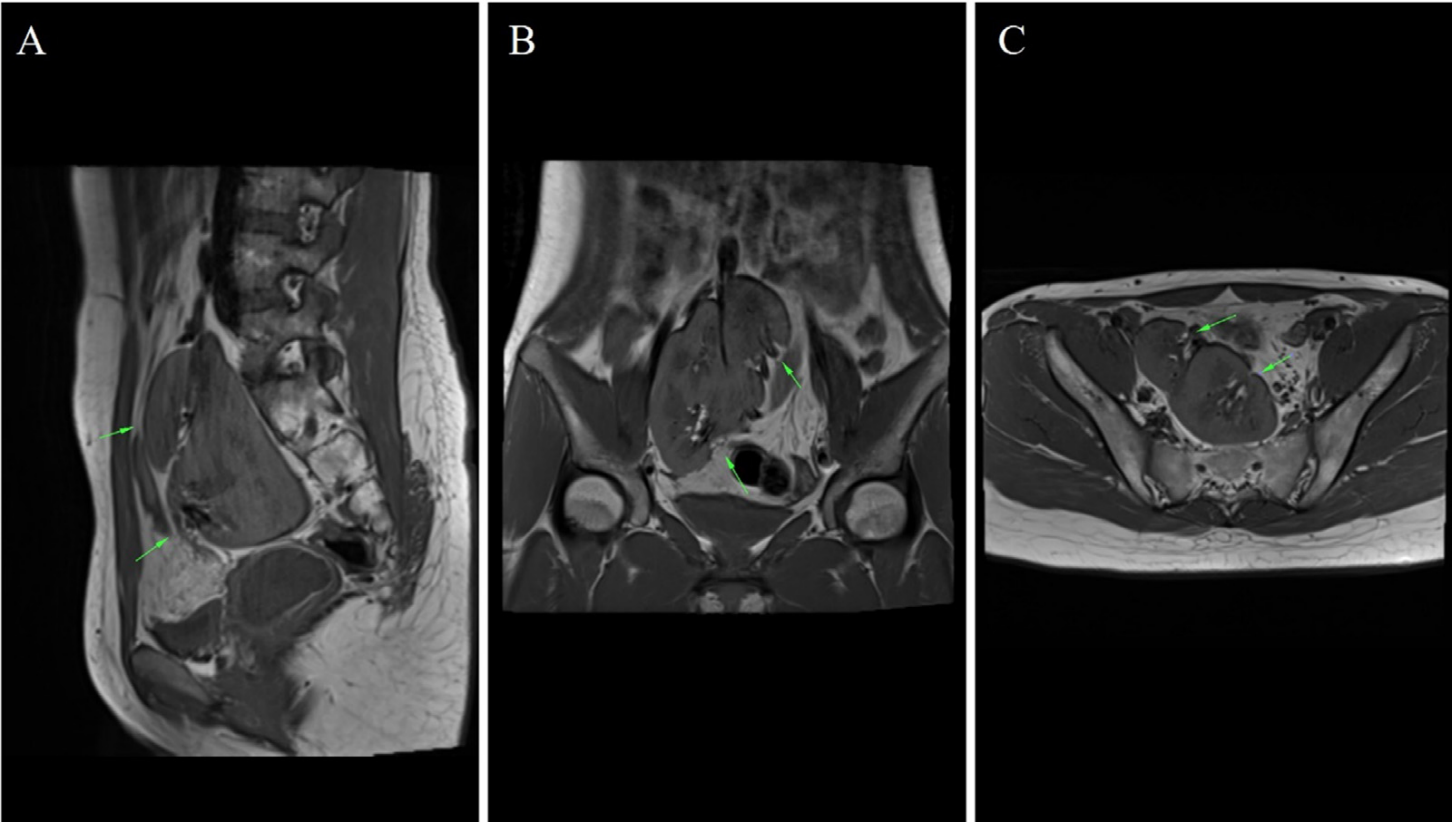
MRI showed cross-fused ectopic kidney within the pelvic cavity on sagittal (A), coronal (B), and transverse (C) section. Two renal pelvis was found (green arrow).
